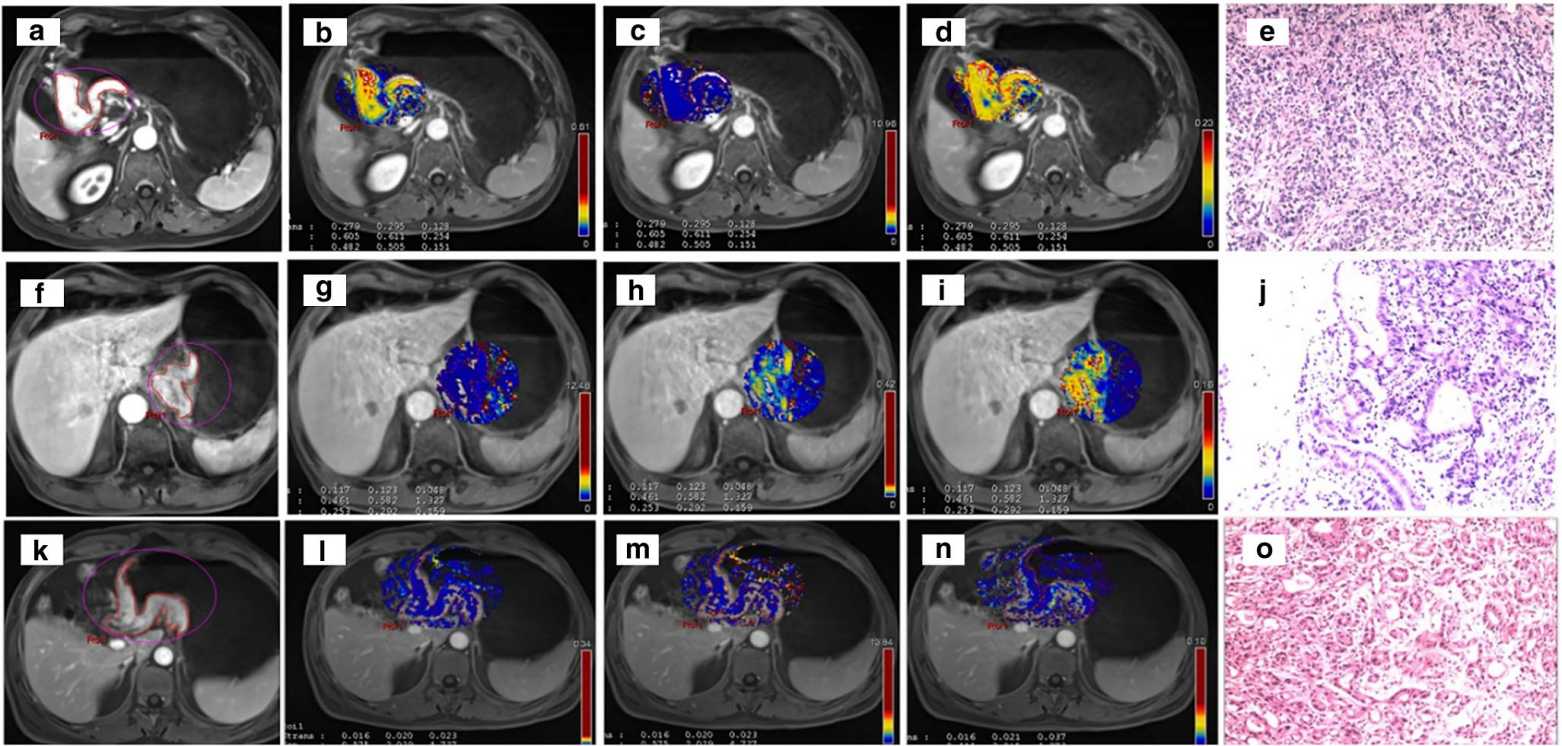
DCE-MRI showing a tumour of the gastric antrum (a) in a 73-year-old male. The K^trans^ (b) was 0.279 min^−1^, the K_ep_ (c) was 0.605 min^−1^ and the V_e_ (d) was 0.482. Final pathology (e): diffuse type (Lauren classification), staged as pT4aN3. DCE-MRI of a tumour of the gastro-oesophageal junction (Siewert III) (f) in a 68-year-old male. The K^trans^ (g) was 0.117 min^−1^, the K_ep_ (h) was 0.461 min^−1^ and the V_e_ (i) was 0.253. Final pathology (j): mixed type (Lauren classification), staged as pT3N1. DCE-MRI of a tumour of the gastric antrum (k) in a 49-year-old male. The K^trans^ (l) was 0.016 min^−1^, the K_ep_ (m) was 0.575 min^−1^ and the V_e_ (n) was 0.029. Final pathology (o): intestinal type (Lauren classification), staged as pT4aN2
